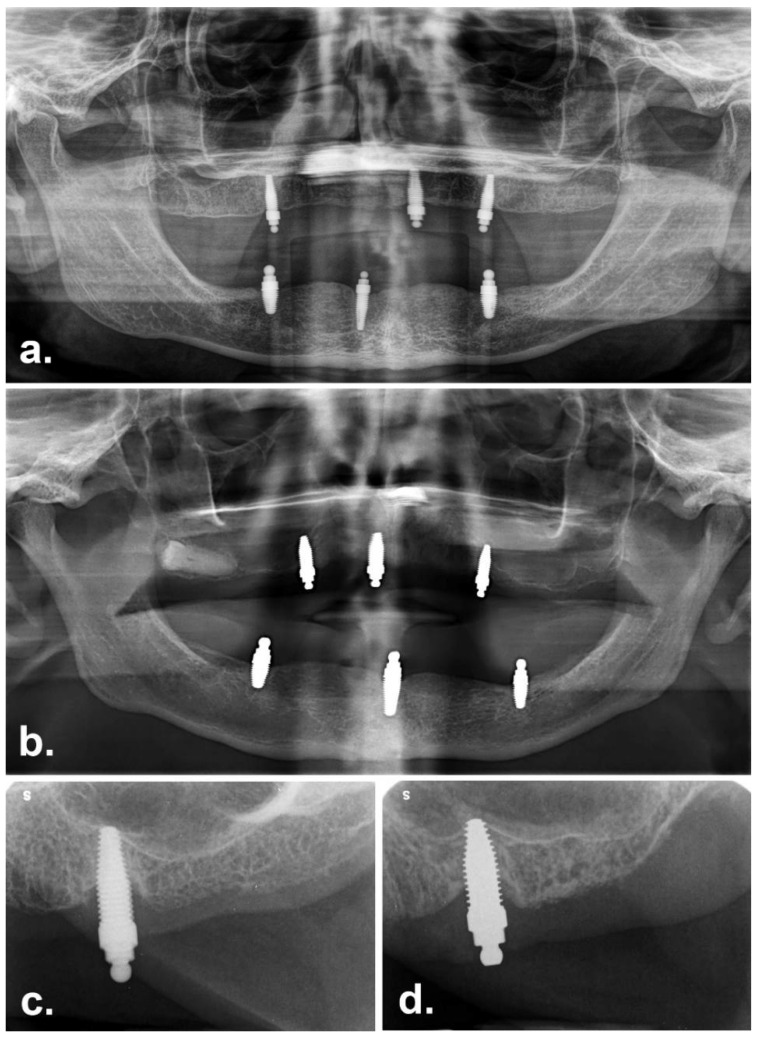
Representative radiographs from the recalled participants: (a) Orthopantomogram of participant with Ti implants (clinical images in Figure 4) (b) Orthopantomogram of participant with ZC implants (clinical images in Figure 4) (c) intraoral peri-apical radiograph showing peri-implant bone loss around a Ti implant in the maxilla (d) intraoral peri-apical radiograph showing peri-implant bone loss around a ZC implant in the maxilla.
